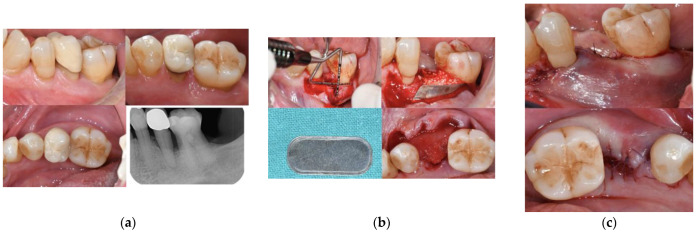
(a) Preoperative buccal, occlusal, lingual clinical views and x-ray showing a compromised left mandibular second premolar. (b) Surgical procedure involving atraumatic extraction of the tooth, Mg-membrane positioning at the buccal site, and bone grafting of the socket. Occlusal seal using CDM stabilized with PGA 7-0 sutures. (c) Occlusal and buccal view of flap stabilization through PGA 6-0, aiming to reach primary wound closure.
